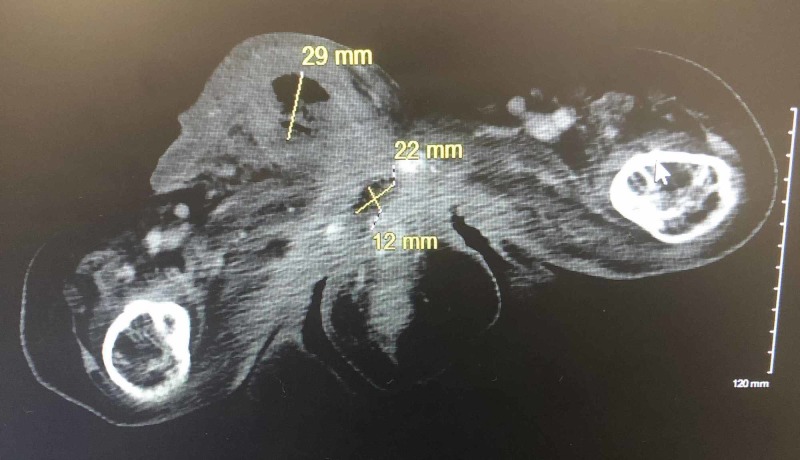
Demonstration of corpus cavernosum abscess with secondary fluid collection on computed tomography (CT).
